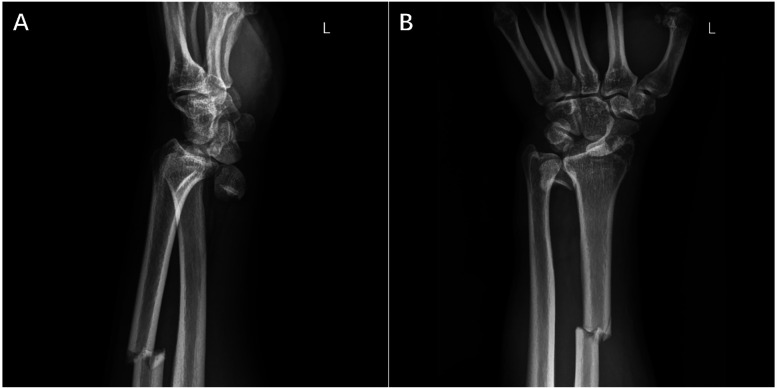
Pre-operative radiography nnshowed the injured left forearm. Lateral (A) and anteroposterior (B) radiographs of the left forearm showed dislocation of lunate and scaphoid as well as multiple radial fractures.
